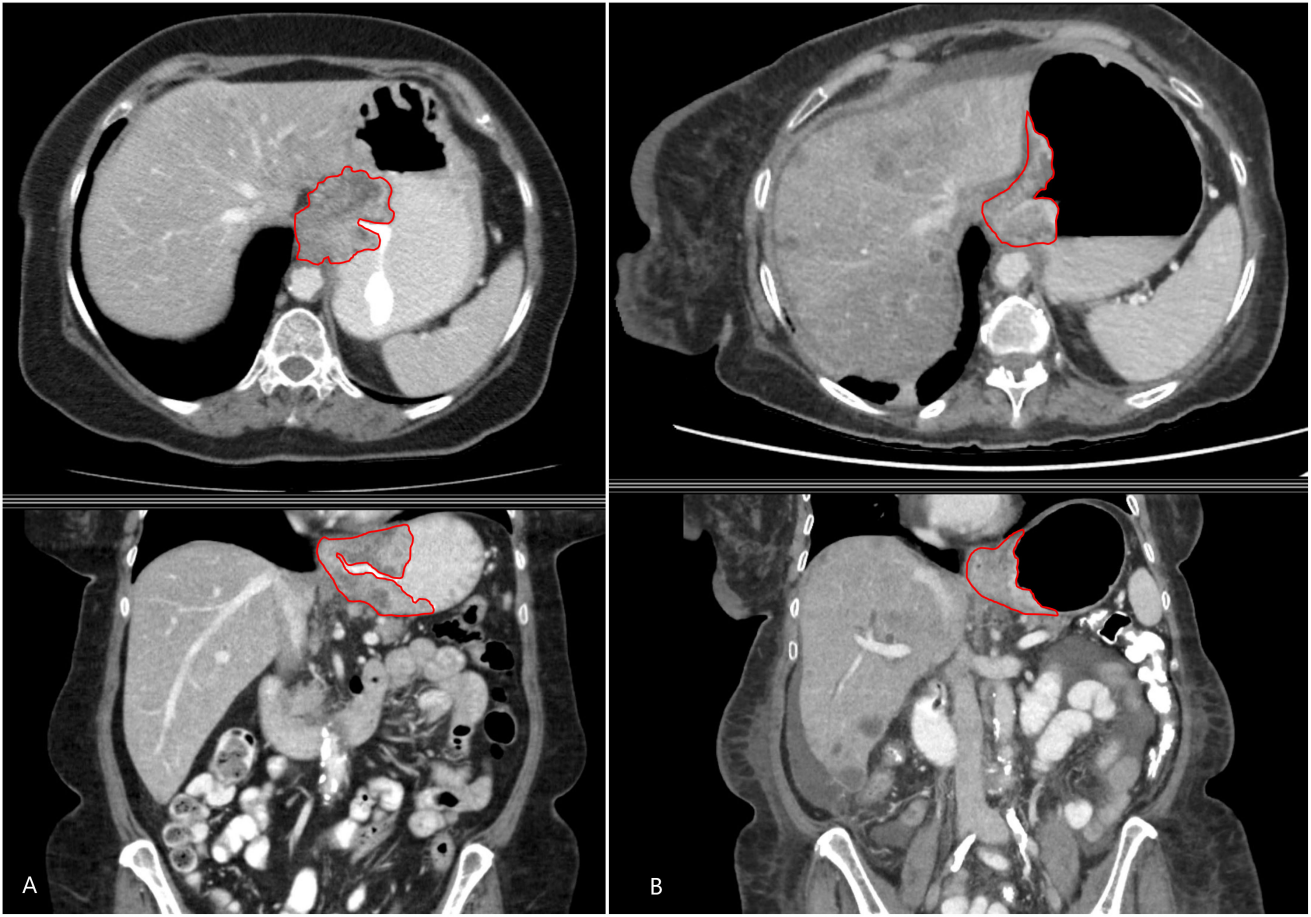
A 48-year-old man with metastatic gastroesophageal adenocarcinoma. Axial (above) and coronal (below) contrast-enhanced CT images showing the primary gastroesophageal adenocarcinoma and the contours of segmentation on the (A) baseline and (B) treatment/follow-up (8–12 weeks on chemotherapy) CT.
